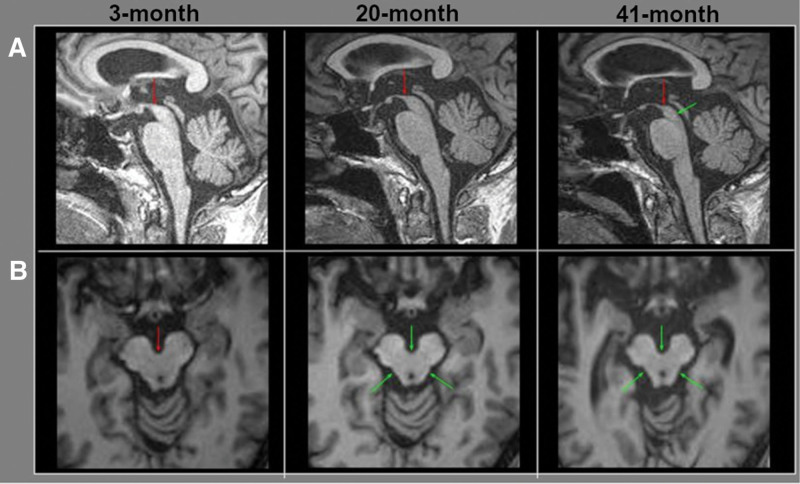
MR images of midbrain in a progressive supranuclear palsy patient. (A) Sagittal T1-weighted image. (B) Axial T1-weighted image. On the 3-month MRI, there were minimal and subtle changes in the midbrain (red arrows). Subsequently, flattening of the superior surface of the midbrain was apparent (red arrow), and the AP diameter at the level of interpedencular groove was slightly decreased (green arrows) on 20-month MRI. Progressive flattening (red arrow) and reduction of the volume (green arrows) was remarkable on 41-month MRI. MRI = magnetic resonance imaging. AP = anteroposterior.
